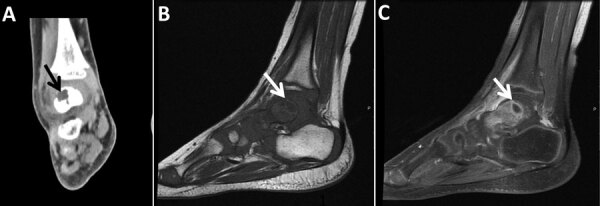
Imaging of the left ankle for a 3-year-old boy (case 3) with Q fever osteoarticular infection, Israel. A) Computed tomography imaging, coronal view, shows a lytic lesion in the talus (black arrow). B, C) Magnetic resonance imaging sagittal T1 (B) and sagittal T1 fat saturation + contrast (C) demonstrate a lesion in the posterior aspect of the talus (white arrows), determined to be an intramedullary abscess (Brodie’s abscess) surrounded by edema.
